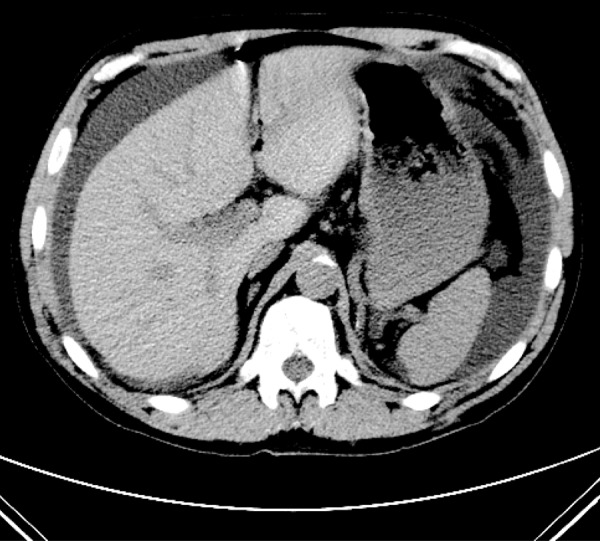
Axial, noncontrasted CT image at the level of the porta hepatis demonstrates freely mobile peritoneal fluid tracking along the liver, spleen, and left lateral abdominal wall at the initial diagnosis of pseudomonas peritonitis.
